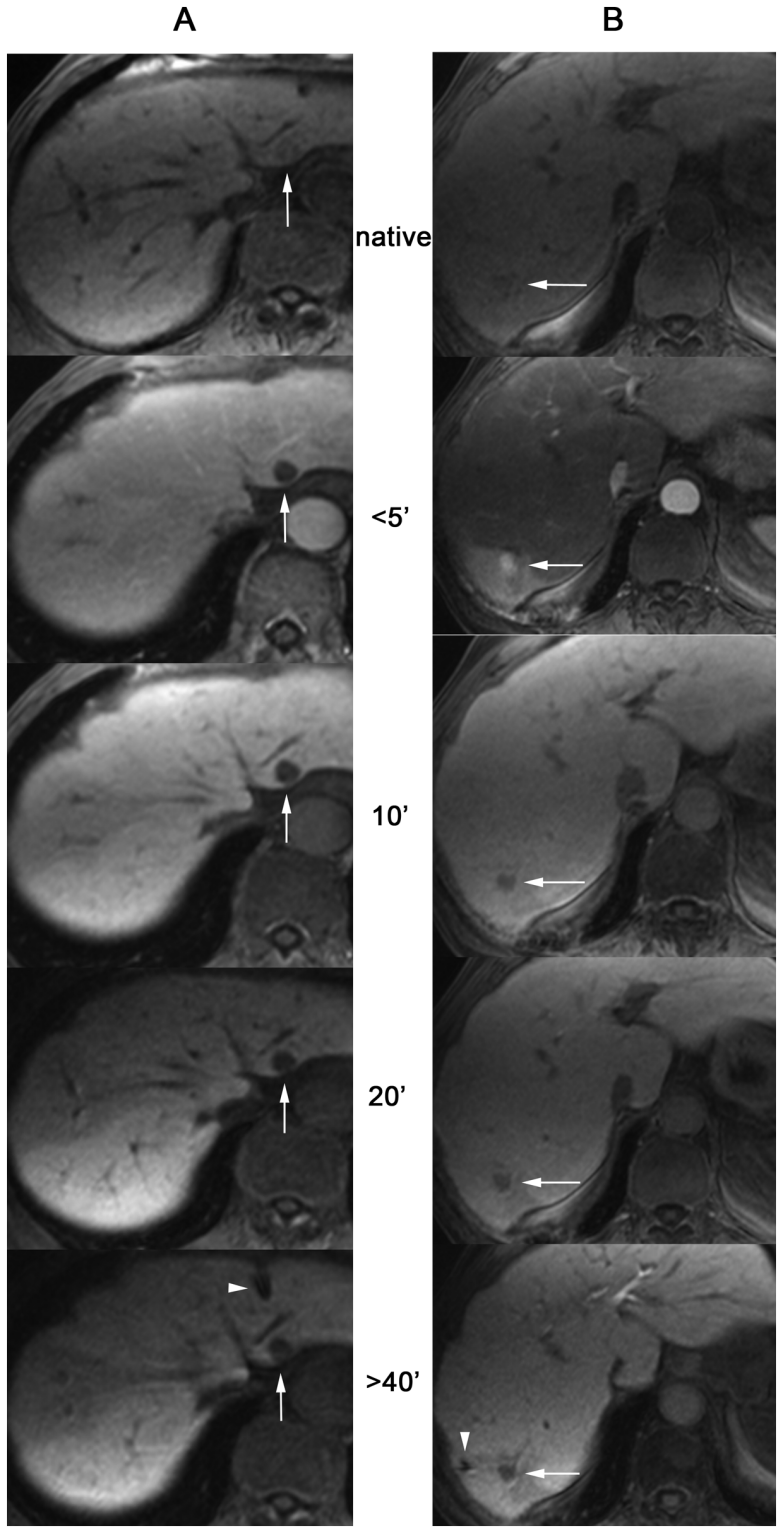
Liver-to-tumor contrast promotes planning and placement of applicators. Dynamic imaging (breath-hold T1-weighted GRE FLASH 3D sequences) of both non-enhancing Tneg^G1^ (A) and enhancing Tpos^G1^ (B) during planning and catheter placement phase in two different cases. Column A shows a sustainable high-low contrast between liver parenchyma and target tumor (arrow), whereas column B shows a transient low-high contrast between parenchyma and tumor (arrow). Advantageous target outlining at late phase initiates catheter (arrow heads) approach in both cases.
